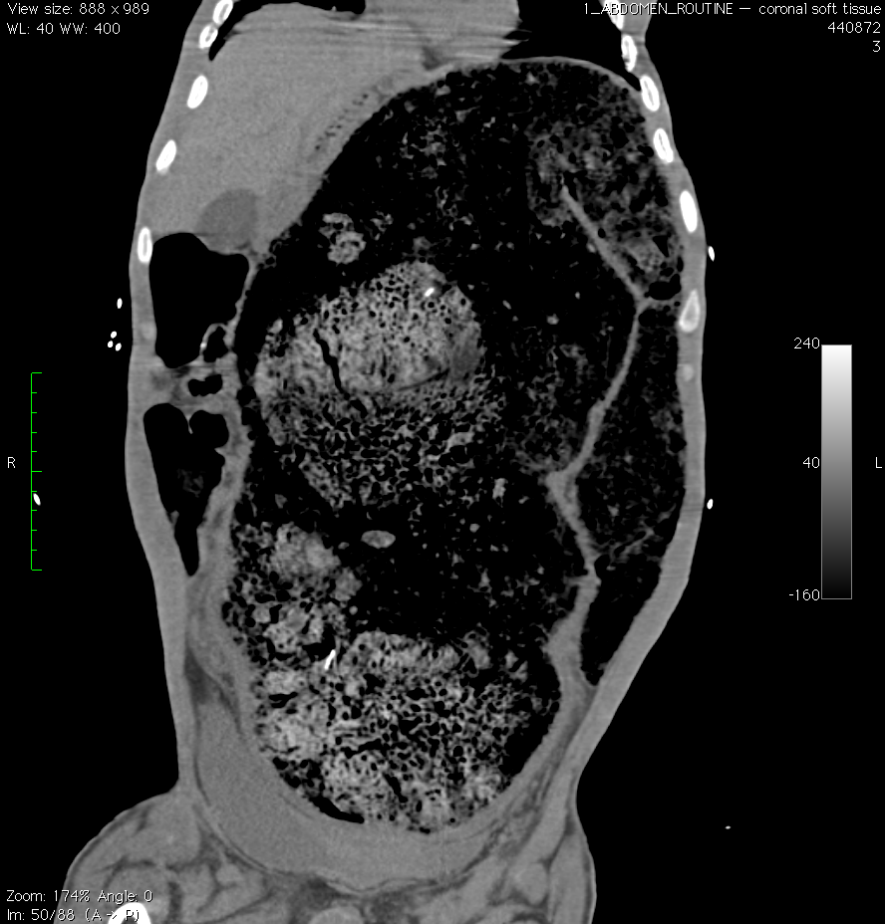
Coronal CT image of pseudo-obstruction and severely dilated colon that almost completely fills the view of the abdomen cavity.
